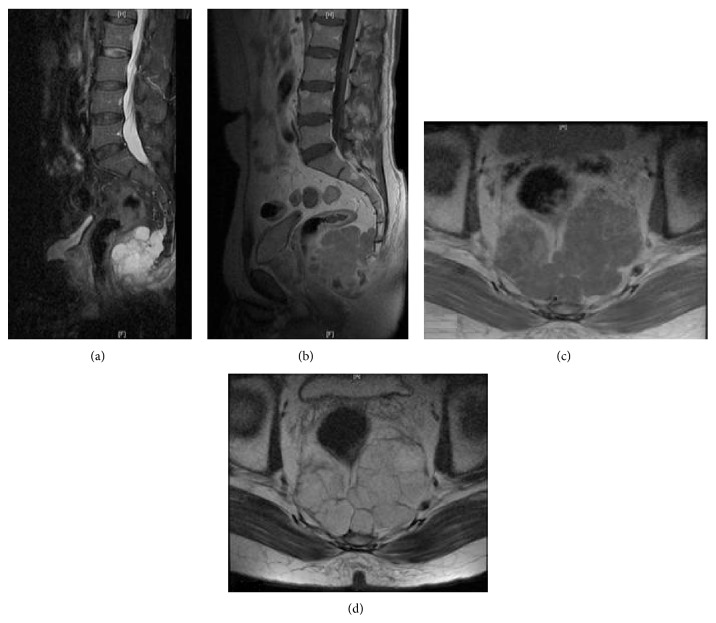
Preoperative sagittal and axial MRI of sacrococcygeal chordoma.
